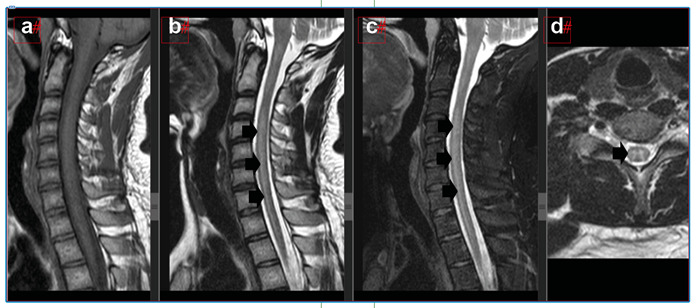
MRI cervical spine in sagittal T1 (a), sagittal T2 (b), sagittal short tau inversion recovery (STIR) (c), and axial T2 (d). Images demonstrate a longitudinally extensive hyperintense signal (b, c, and d - arrows). Suggestive of longitudinally extensive transverse myelitis.
